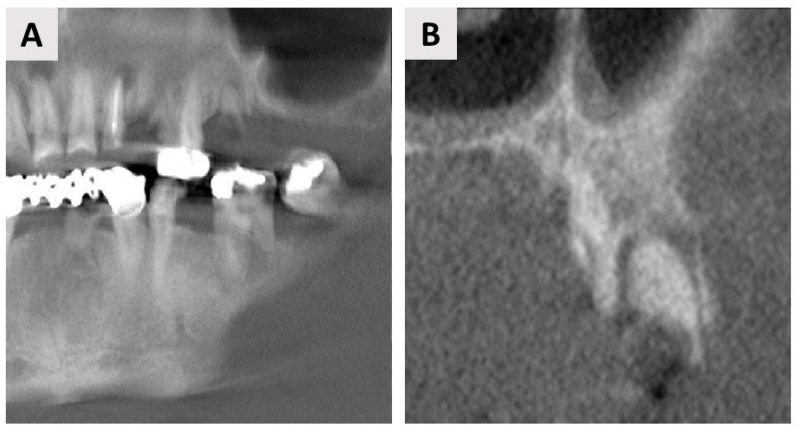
(A) A panoramic CBCT section shows the associated apical radiolucency of tooth 25 (FDI notation system). (B) The coronal CBCT section shows a closer look at tooth 25, which has a very thin buccal wall.
